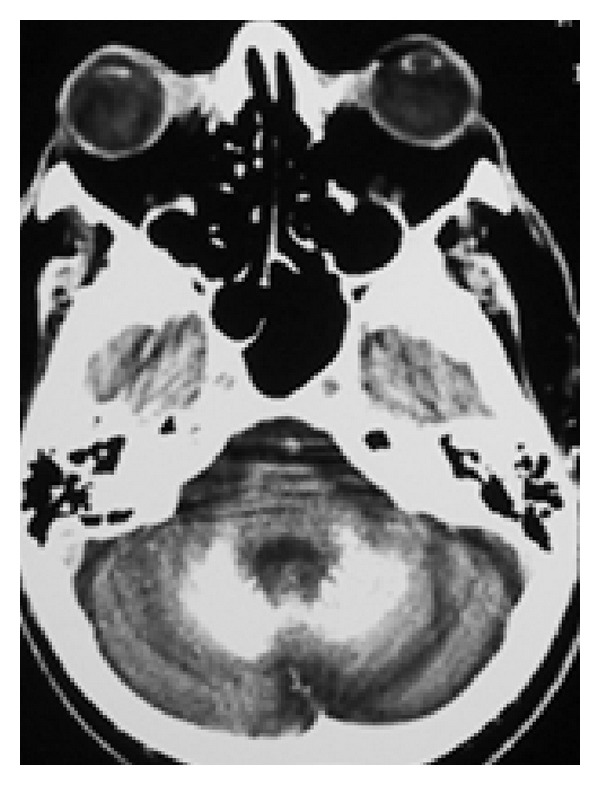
CT scan axial view demonstrating calcifications in both cerebellar hemispheres.
